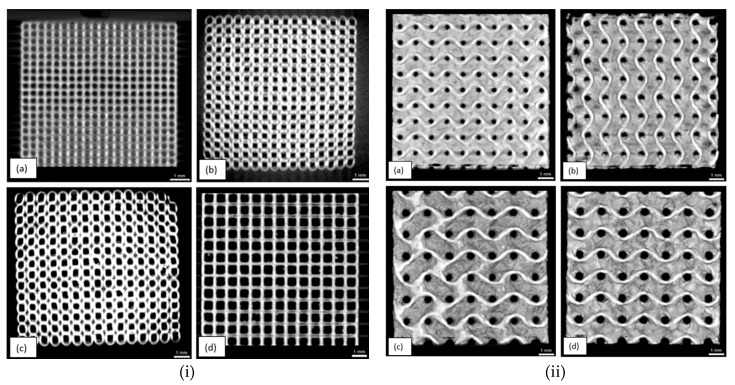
CT Image of (i) cube and (ii) gyroid sample: (a) 0.3 mm; (b) 0.4 mm; (c) 0.5 mm; and (d) 0.6 mm.
